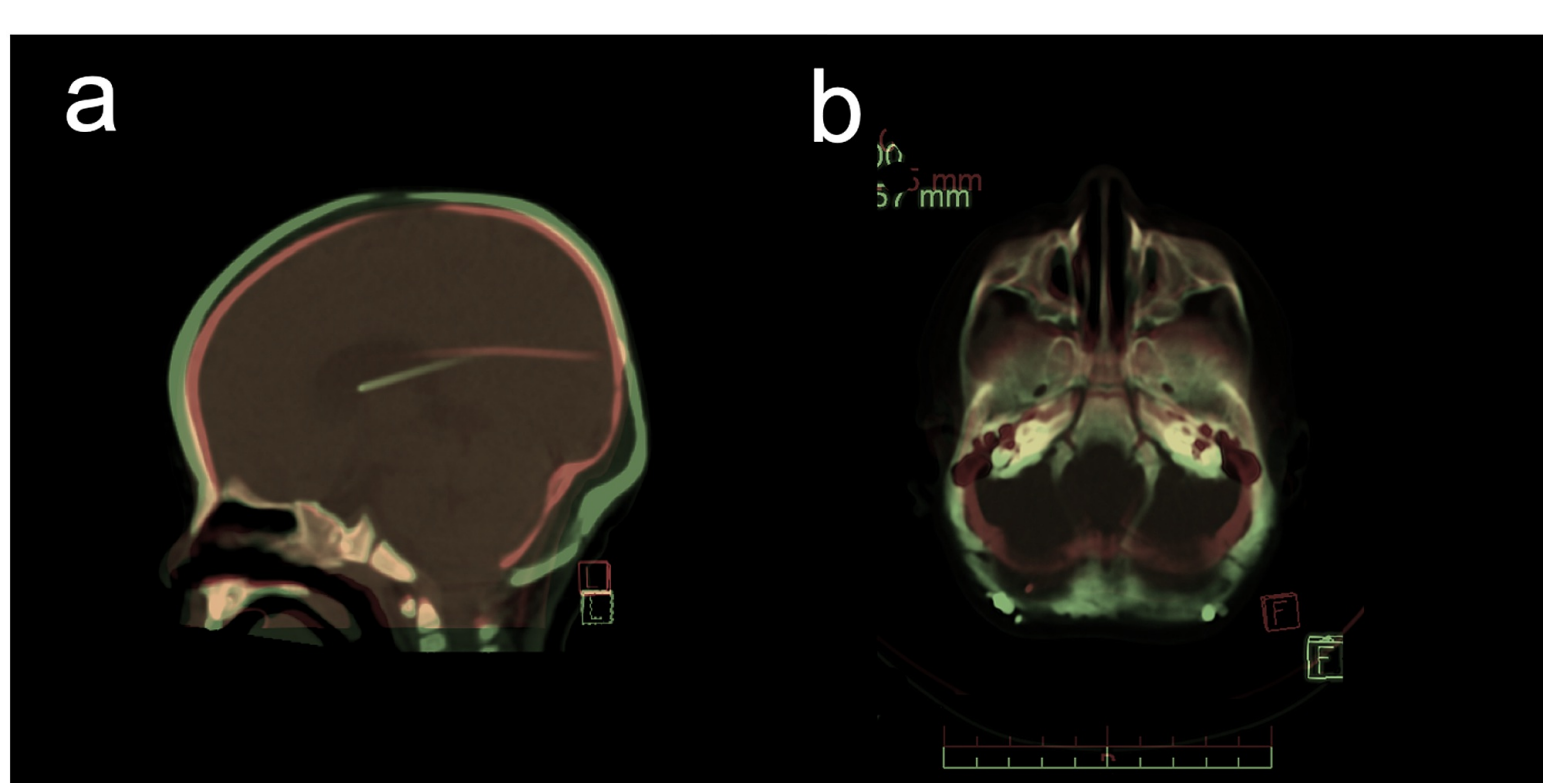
Computerized tomography scan overlay The extent of change to the posterior fossa after distraction can be appreciated in the saggital CT (a) and axial CT overlay (b) shown. Pre-op CT is shown in 'red',  and post-distraction is shown in 'green'.
